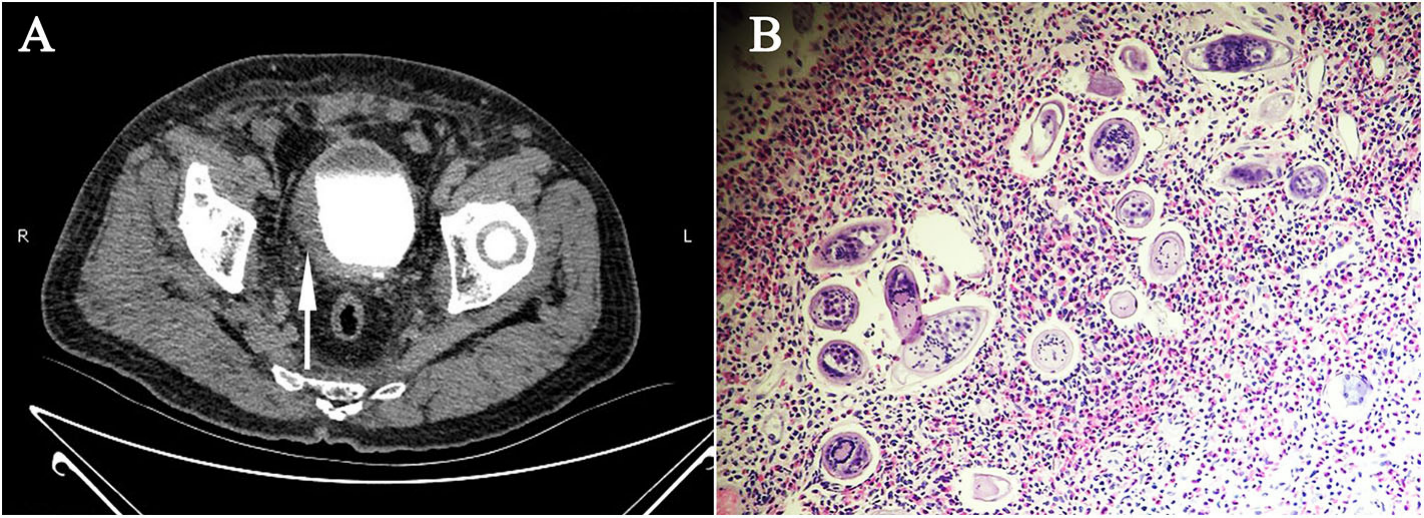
Radiological features and parasitological identification of patients with infections of *Schistosoma haematobium*. (A) CT urography showing the thickened and stiff wall of the bladder (pointed arrow) of a patient with chronic infection of *S. haematobium*. (B) Vesical biopsy tissue section showing schistosomal eosinophilic granuloma, with *S. haematobium* eggs observed (×400).
